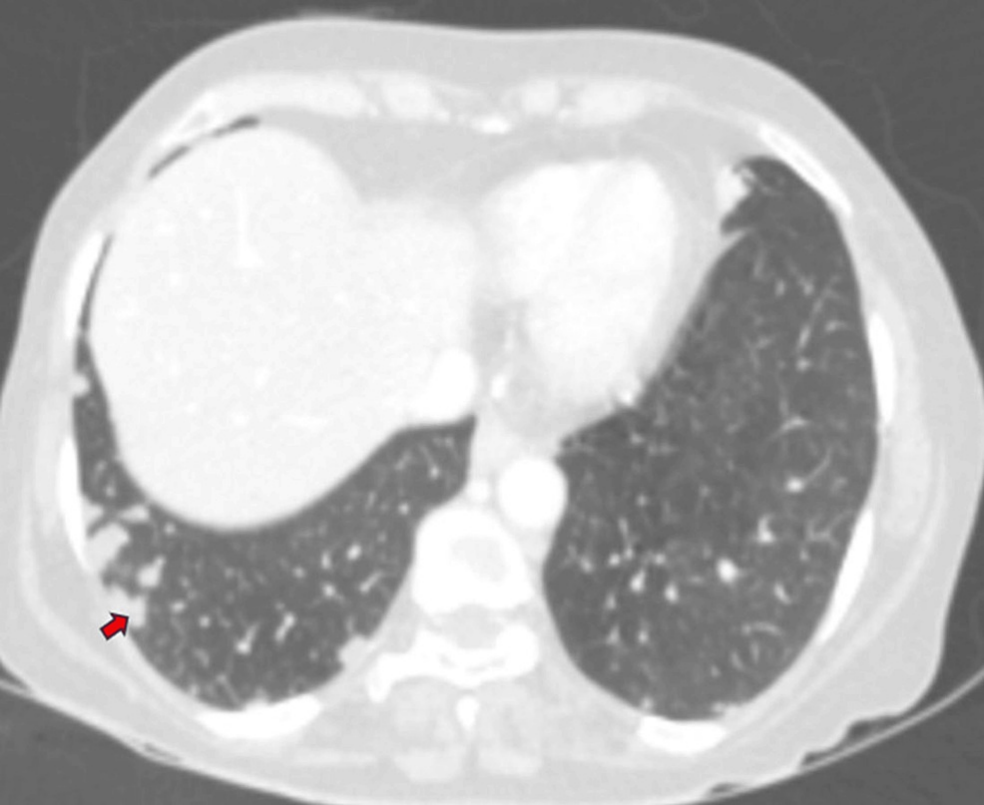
CT axial lung window image of the base of thorax showing multiple well-defined subpleural pulmonary nodules (red arrow). CT: Computed tomography
